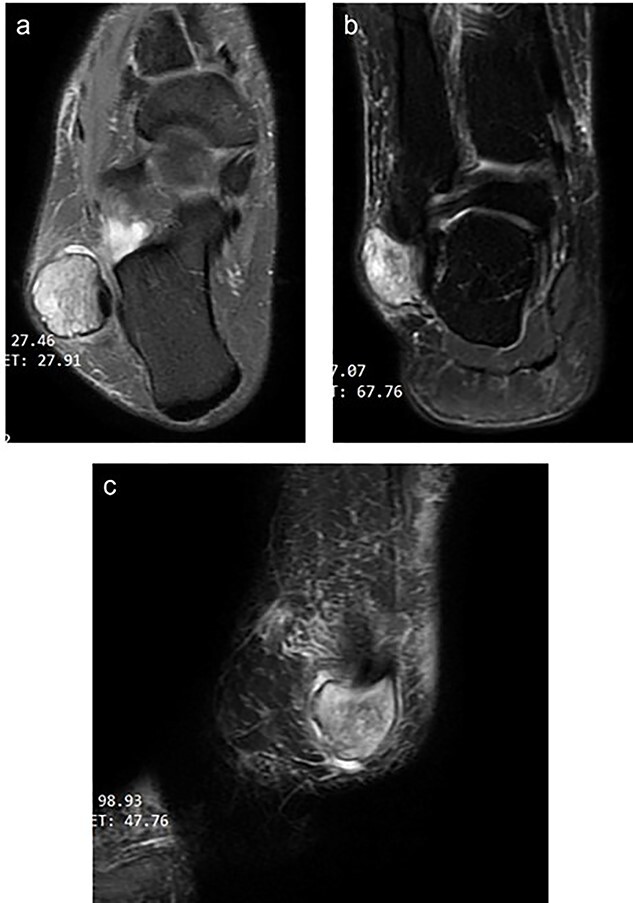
Magnetic resonance image of the right ankle (a: T2-weighted axial view b: T2-weighted coronal view c: fat-saturated T2 weighted sagittal view) showing a thin-walled well-circumscribed lesion within the peroneus longus tendon.
